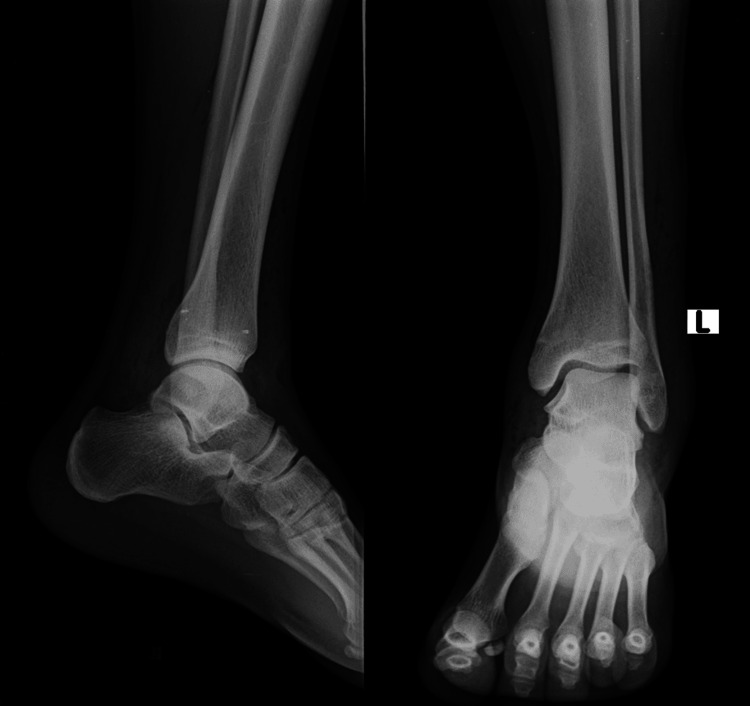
Plain radiographs (anteroposterior and lateral views) demonstrating the presence of air pockets localized within the subcutaneous tissues surrounding the ankle and extending into the distal leg
